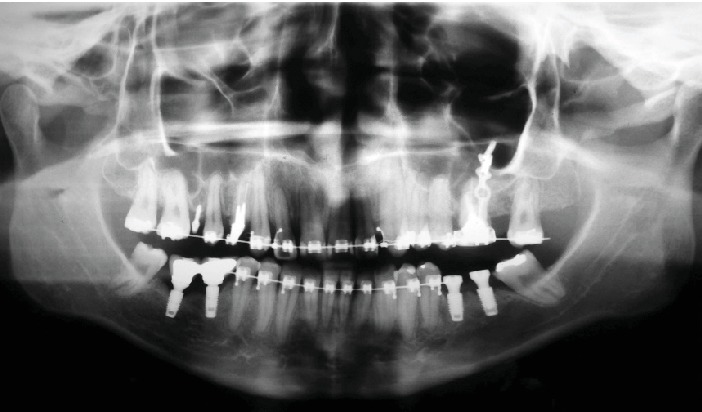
Progress panoramic radiograph.
